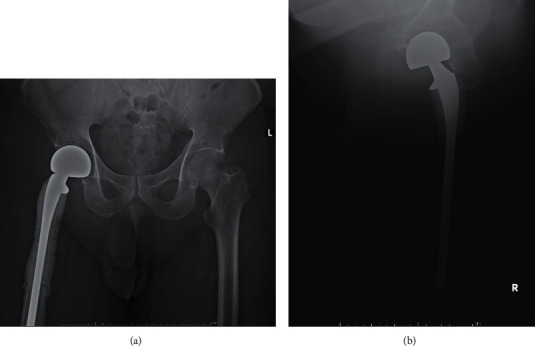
Postoperative radiographs after en bloc resection and reconstruction of the proximal femur: anteroposterior view (a) and trans inguinal view (b).
